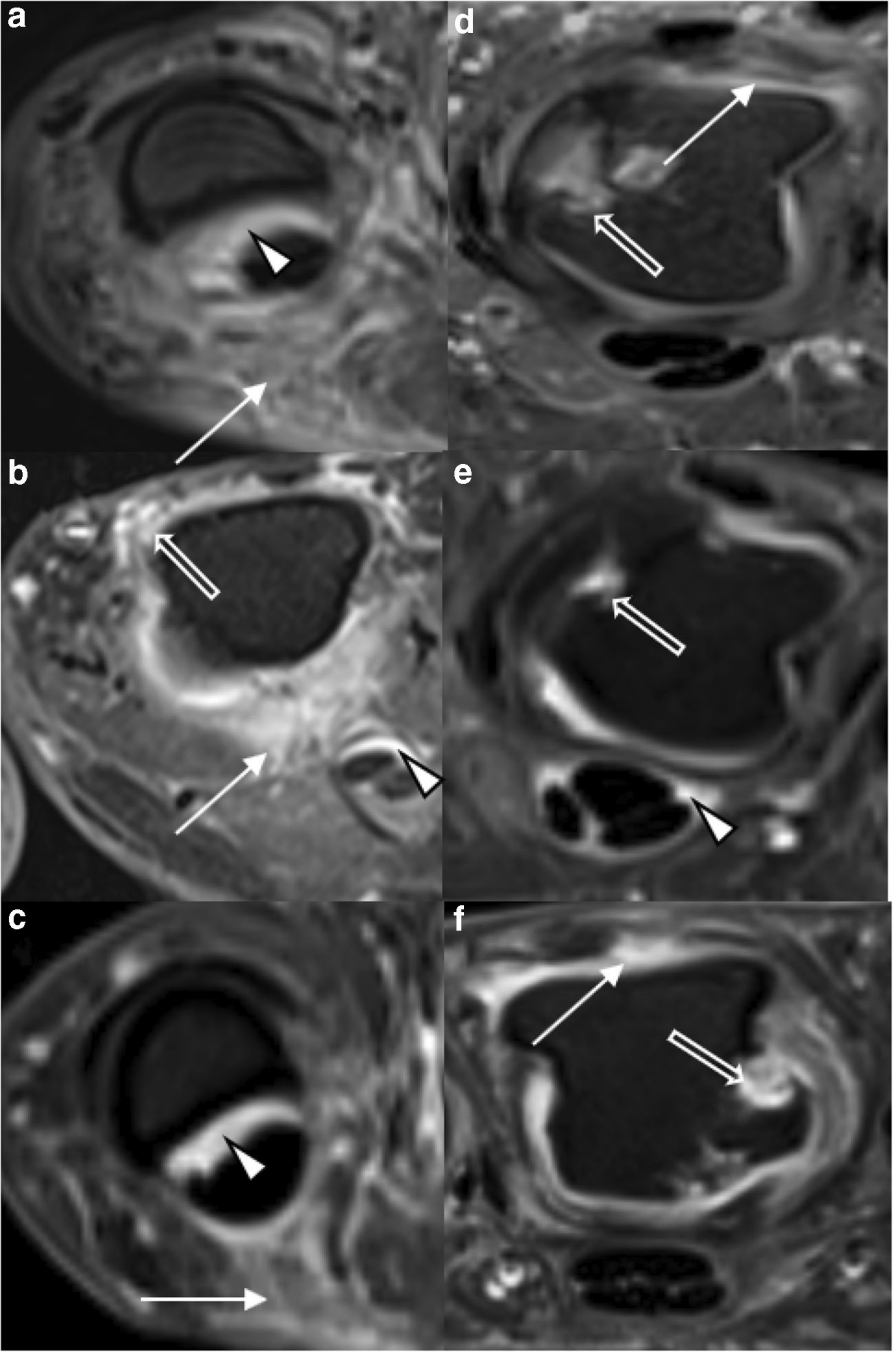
Detailed view of representative MRI findings in psoriatic arthritis (PsA) and rheumatoid arthritis (RA). Transversal T1w fat-saturated contrast-enhanced sequences of selected MCP joints of three patients each with PsA (a–c) and RA (d–f). a 29-year-old female. Severe periarticular inflammation (white arrow) with additional flexor tenosynovitis (arrowhead). b 47-year-old female. Severe periarticular inflammation (white arrow) with synovitis (open arrow) and flexor tenosynovitis (arrowhead). c 37-year-old male. Moderate periarticular inflammation (white arrow) with corresponding flexor tenosynovitis (arrowhead). d 55-year-old male. Widespread synovitis (white arrow) and multiple bone erosions (arrowhead). e 48-year-old female. Bone erosion (open arrow) and slight flexor tenosynovitis (arrowhead). f 39-year-old male. Bone erosion (open arrow) and synovitis (white arrow). Note the absence of periarticular inflammation in d–f despite significant inflammatory joint changes at the joint level
